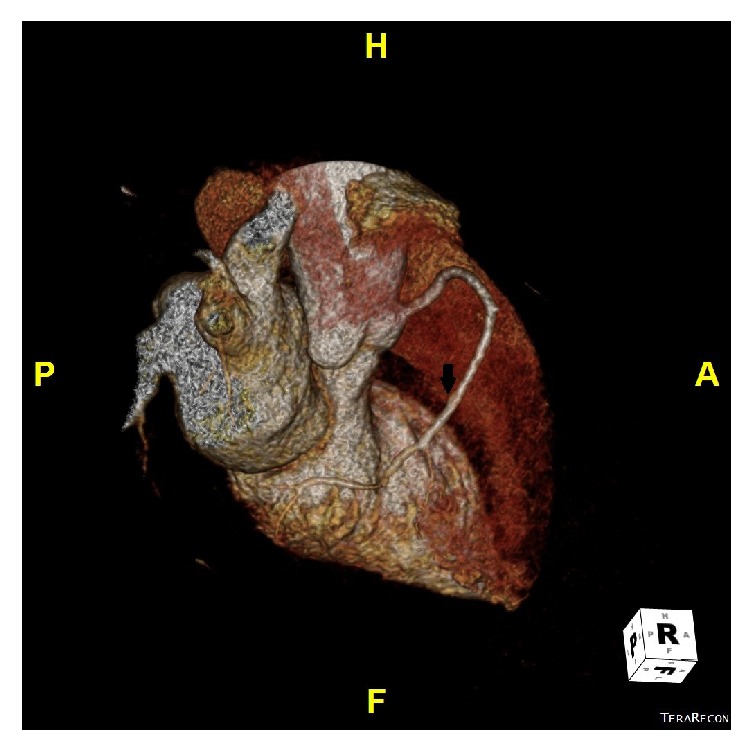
Inferior view three-dimensional computed tomography scan showing large dominant right coronary artery (arrow).
